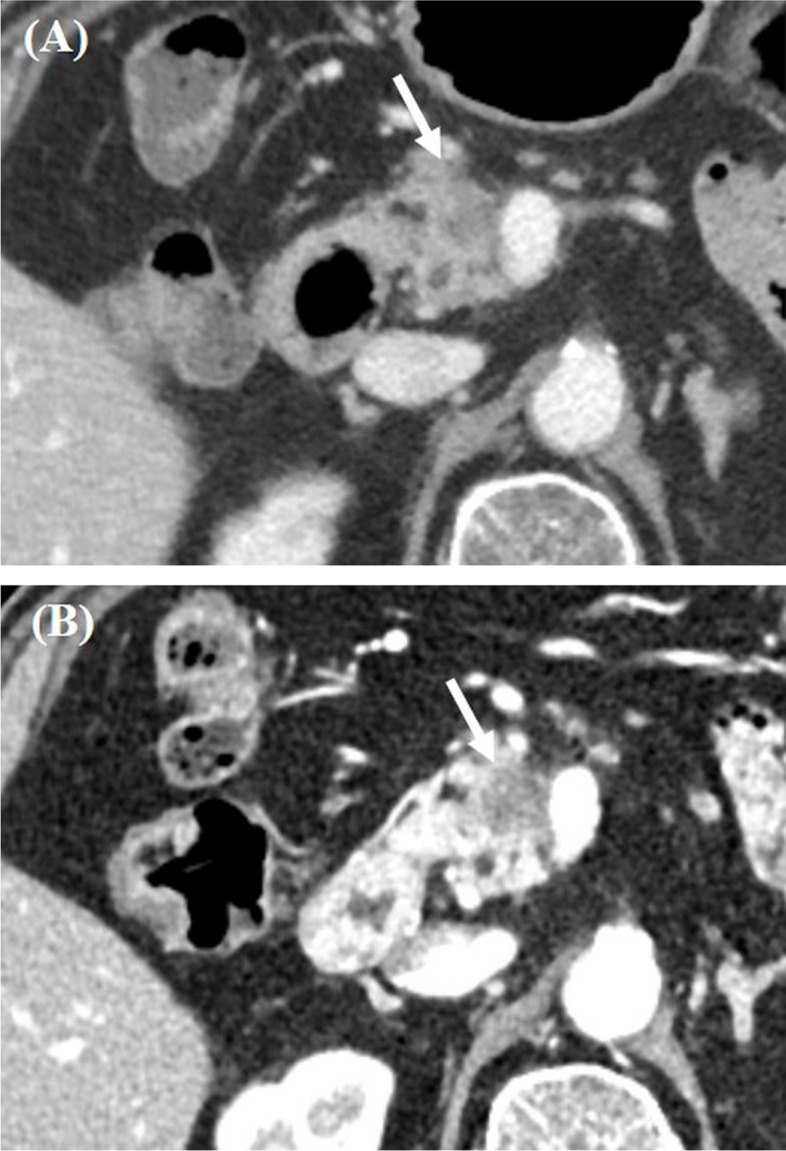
CT images obtained from a 79-year-old man with pancreatic ductal adenocarcinoma in the pancreas head. A Arterial phase axial contrast enhanced CT image (window width, 400HU; window level, 40HU) acquired through the standard protocol showed a 1.5 cm hypo-attenuating lesion in the pancreas head (arrow) abutting the SMV. The calculated CNR of pancreatic tumor was 7.4. B Arterial phase axial contrast enhanced CT image (window width, 400HU; window level, 40HU) acquired through the high-resolution protocol also revealed a 1.5 cm hypo-attenuating lesion (arrow) with calculated CNR of pancreatic tumor of 15.7. The abutment of SMV was also noted. Upon reviewing these imaging findings, all three readers classified this patient as having resectable pancreatic ductal adenocarcinoma on both CT protocols. Nevertheless, surgical exploration unveiled unexpected peritoneal seeding, making the tumor unresectable
